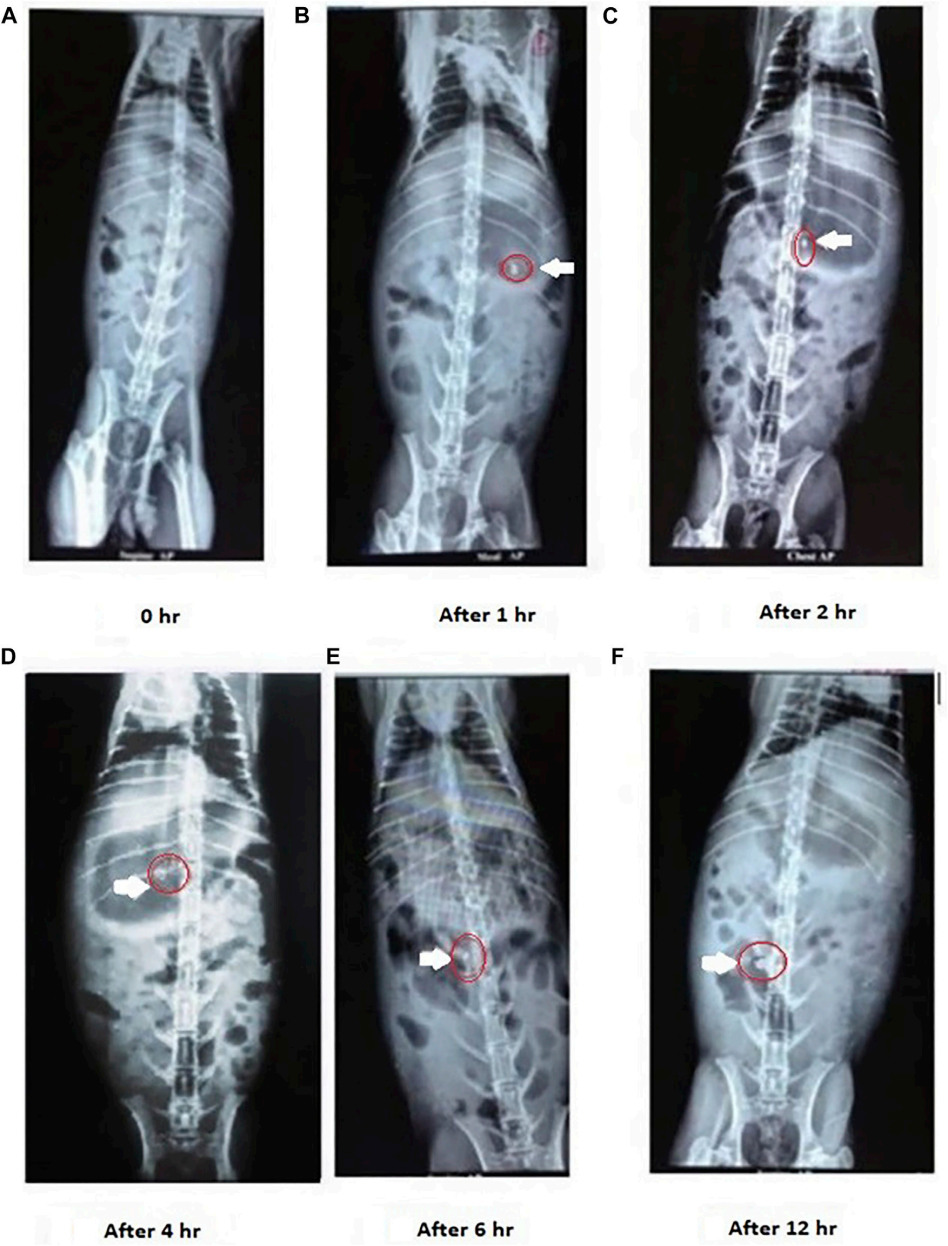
X-ray images of CMN colon targeting studies after administration of M14 mucoadhesive tablet formulation. The tablet remained intact in the gastric and upper intestinal region, On the other hand swelling and distortion in the shape of the tablet were observed in the colonic region.
